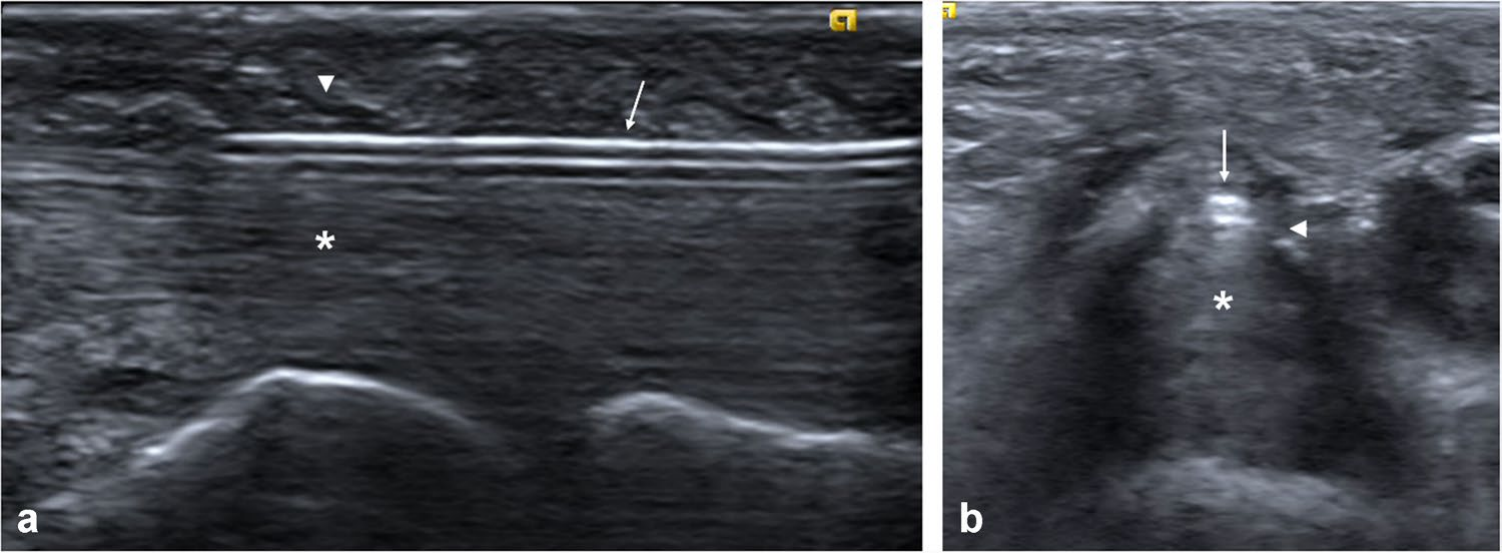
a The fenestration needle (arrow) is advanced to the A1 pulley (arrowhead) from distal to proximal and aligned superficial and parallel to the digit flexor tendons (asterisk (*)) under real-time sonographic guidance using a hockey-stick probe in a longitudinal plane. b Transverse imaging can also be used to ensure fenestration across the entire thickness of the A1 pulley
